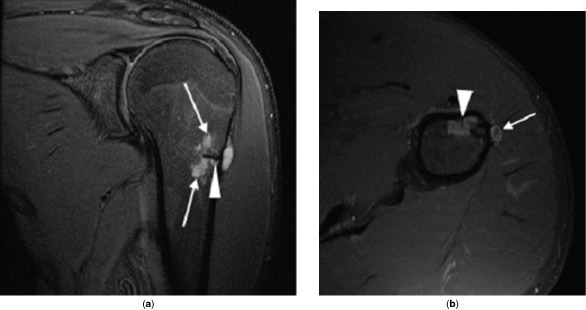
MR images obtained 10 months after those seen in Figure 2, to investigate the complaint of worsened pain. (a) Coronal *T*_2_ fat-saturated image demonstrating the presence of hyperintense intramedullary lobulated mass (arrows) deep to the low signal, linear needle biopsy tract (arrowhead). (b) Axial *T*_1_ fat-saturated post-contrast image demonstrating the original juxtacortical lesion (arrow) as well as cartilage enhancement in the medullary cavity (arrowhead).
